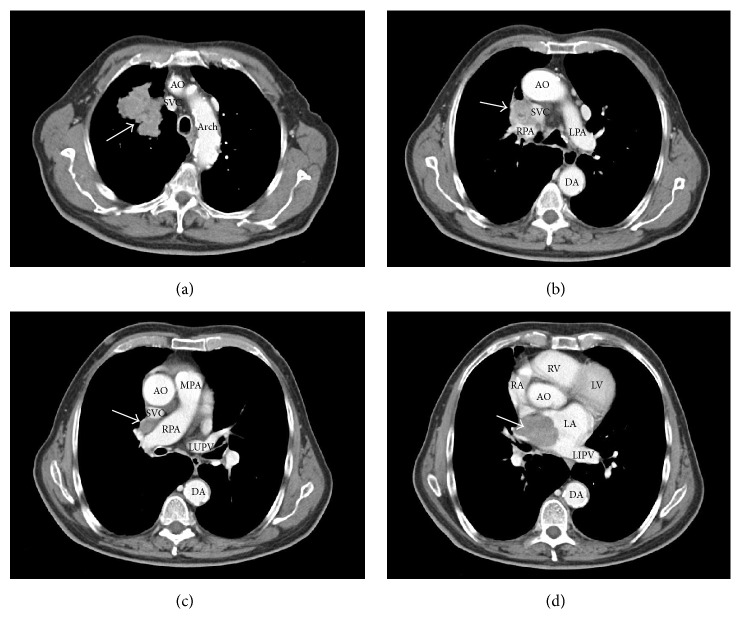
Chest CT showing a large opacity with irregular borders in the right upper lobe (a), with invasion of the right upper pulmonary vein (b-c) and extension into the left atrium (d). The arrows indicate the mass along its pathway from the right upper pulmonary lobe to the left atrium (LA). Note the lack of contrast signal in the right upper pulmonary vein, obstructed by the mass. AO, aorta; DA, descending aorta; LAA, left atrial appendage; LPA, left pulmonary artery; LIPV, left inferior pulmonary vein; LUPV, left upper pulmonary vein; LV, left ventricle; MPA, main pulmonary artery; RA, right atrium; RPA, right pulmonary artery; RV, right ventricle; SVC, superior vena cava.
